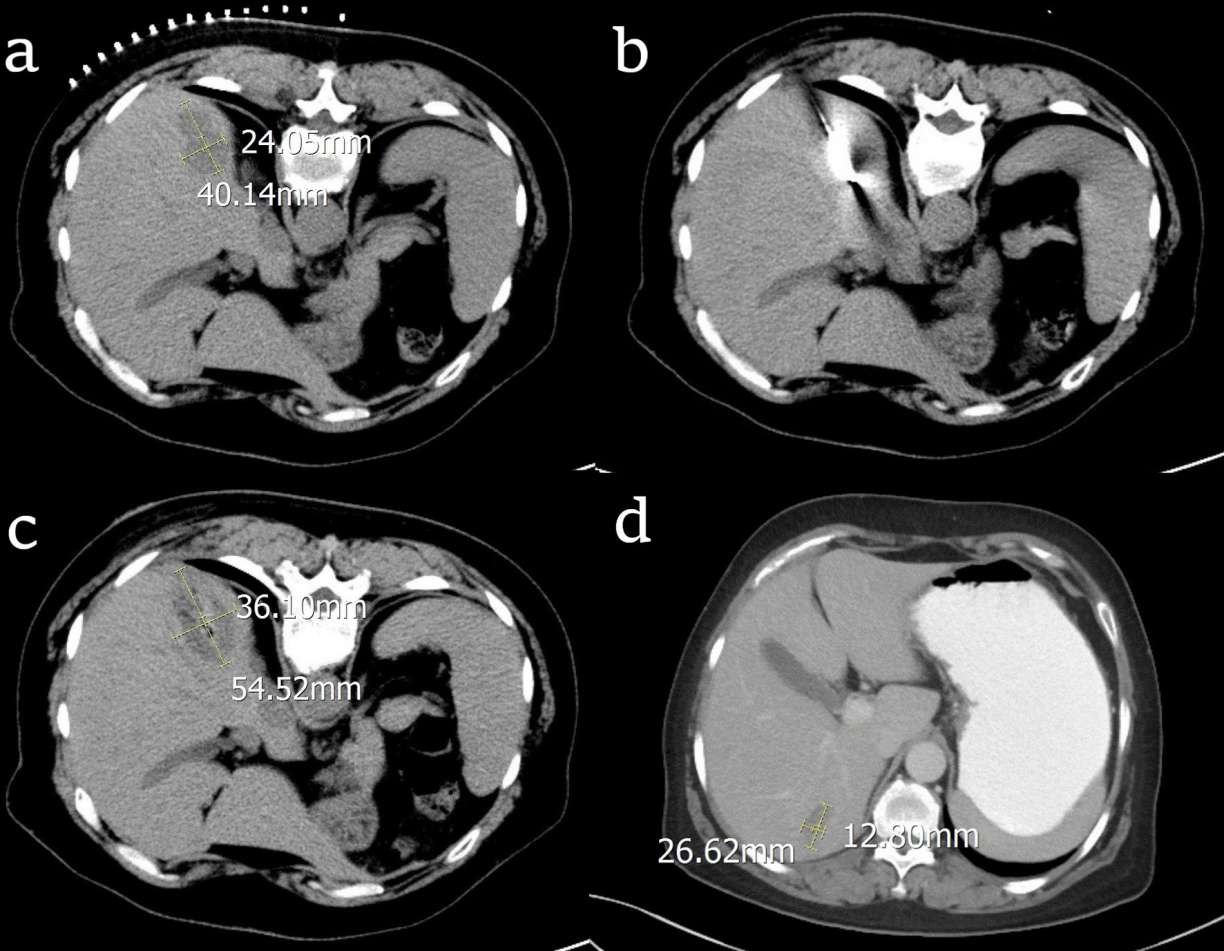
CT images of the process of radiofrequency ablation (RFA) treatment for one patient with ovarian cancer liver metastasis (OCLM). (A) the location and diameter of the tumor before RFA; (B) the radiofrequency electrode in the center of the tumor; (C) the location and diameter of the tumor right after RFA; (D) the location and diameter of the tumor after 6 months.
